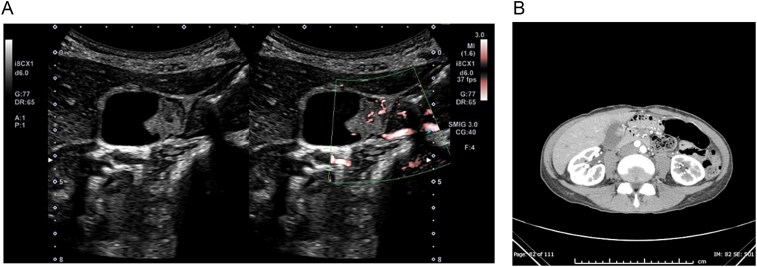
Initial imaging findings. (A) Abdominal ultrasound showing a 17-mm pedunculated polypoid lesion in the gallbladder body with internal blood flow signals. (B) Contrast-enhanced CT demonstrating a gallbladder polyp with enhancement (arrow). No signs of cholecystitis or hemorrhage were observed at this stage.
